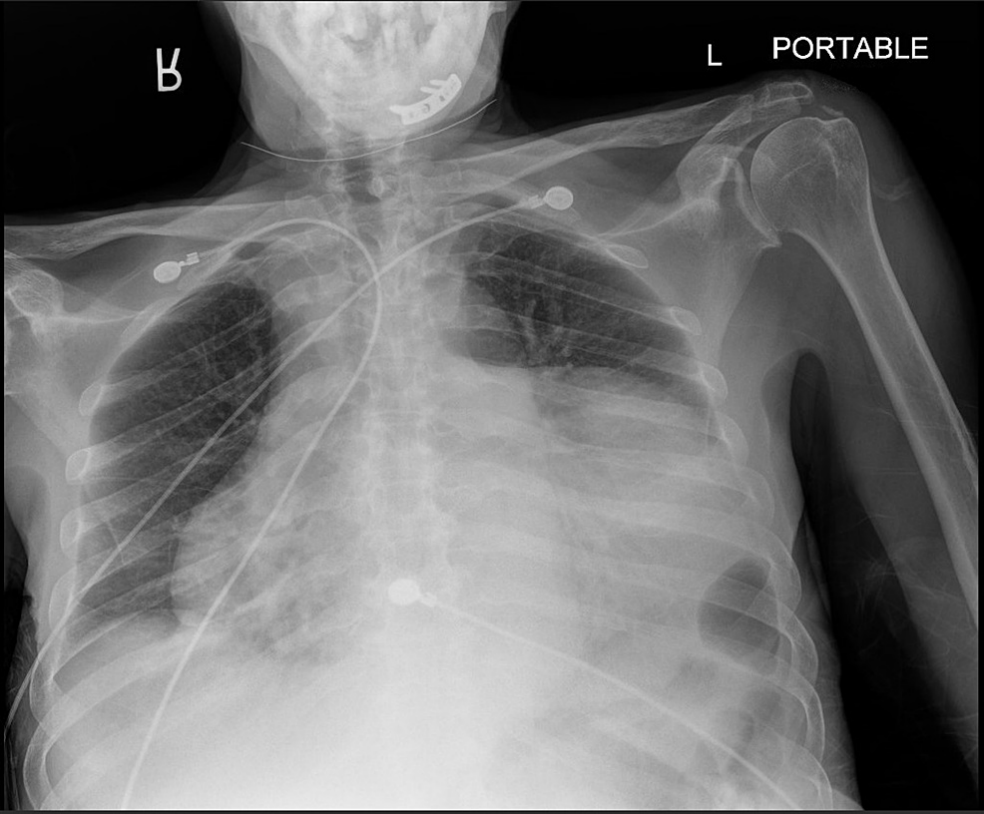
Anteroposterior X-ray of the chest Evidence of a very large pericardial effusion with a classic “water bottle shape” heart.
